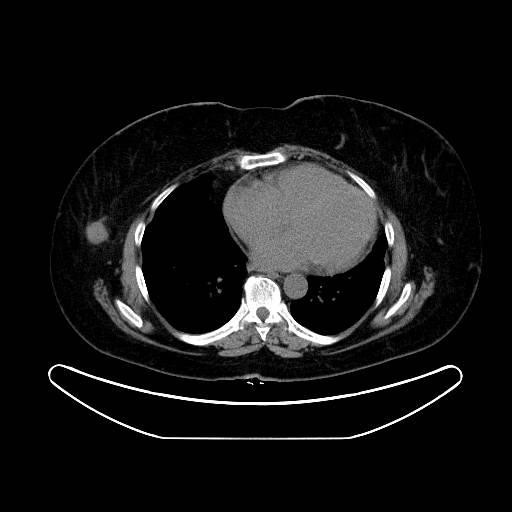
PET/CT scan of the chest showing the mass on the right breast.
